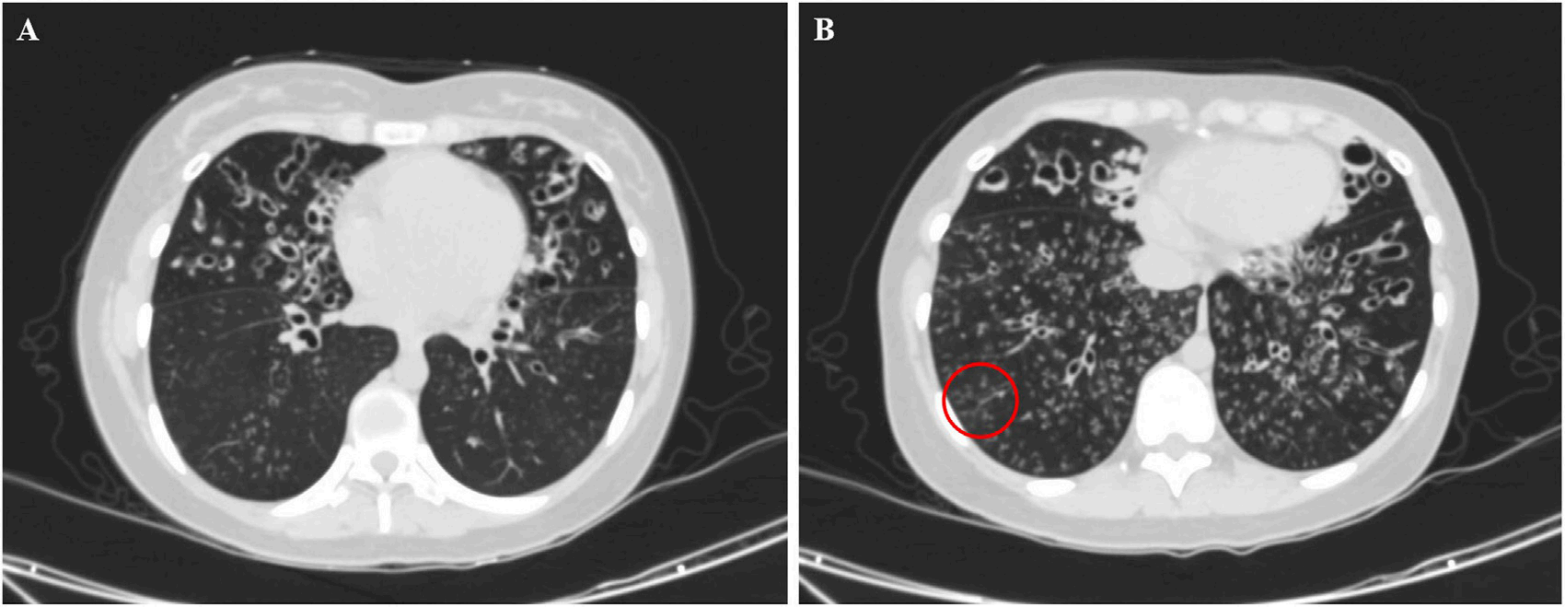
Chest computed tomography image of a 14-year old female patient with PCD on admission (Case 5), showing bronchiectasis in the middle lobe of the right lung and the upper lobe of the left lung (A) with nodular shadows and tree-in-bud signs (B, red circle).
